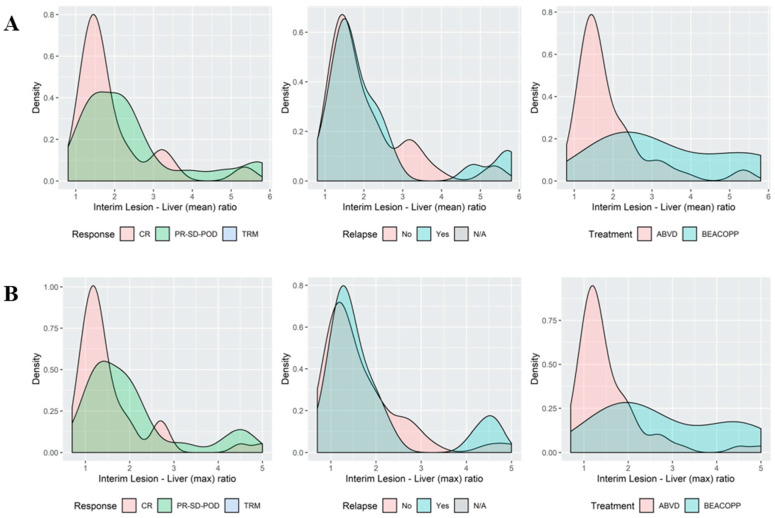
Histograms of the association between the qPET2 thresholds for the LLS ratio and outcomes histogram depicting the occurrence of cases (*Y* axis) at different LLS ratios. The area under the curve represents all cases. Note the overlap in ‘peaks’ at the lower LLS ratio and at the higher LLS ratios. Distribution of the interim lesion SUVmax to liver SUVmean ratio (*X* axis) (A) and the lesion SUVmax to liver SUVmax ratio (B), demonstrating an overlap between patients with ‘good’ (CR; no relapse) and ‘bad’ (PR-SD-POD; relapse) outcomes. For interim PET images and outcomes see Supplementary File S1.
